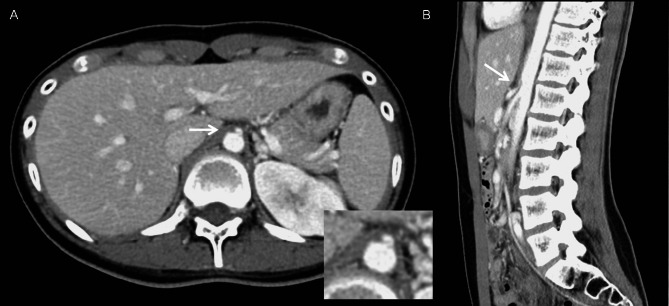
Abdomen-to-pelvis computed tomography with contrast showing the thickened median arcuate ligament and diaphragmatic crura (A, arrow) and the kinked proximal celiac artery, with a hooked appearance, and post-stenotic dilatation (B, arrow)
